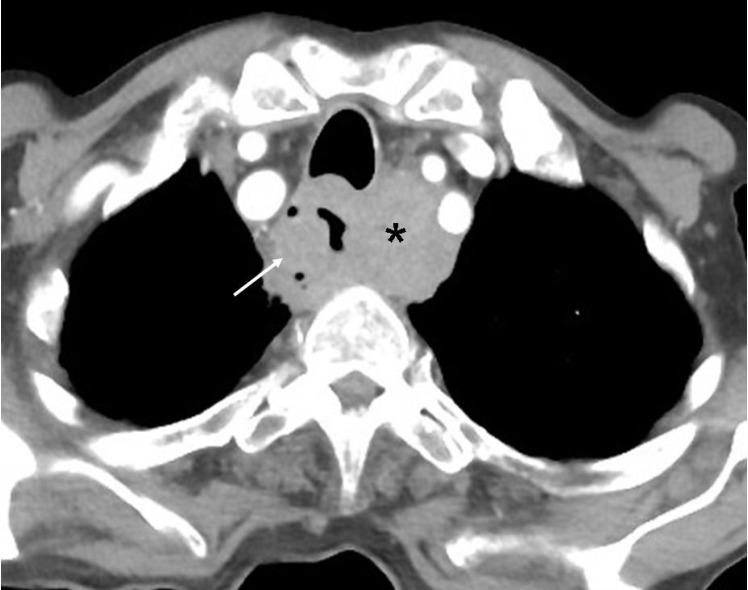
Contrast enhanced CT of the chest of a 58 years old man with carcinoma esophagus shows a large exophytic mass (arrow) in the esophagus narrowing its lumen and a large lymph nodal metastasis (asterisk) in the left paraesophageal region. Small lucencies within the mass suggest necrotic changes. Note anterior displacement of the trachea by the mass.
